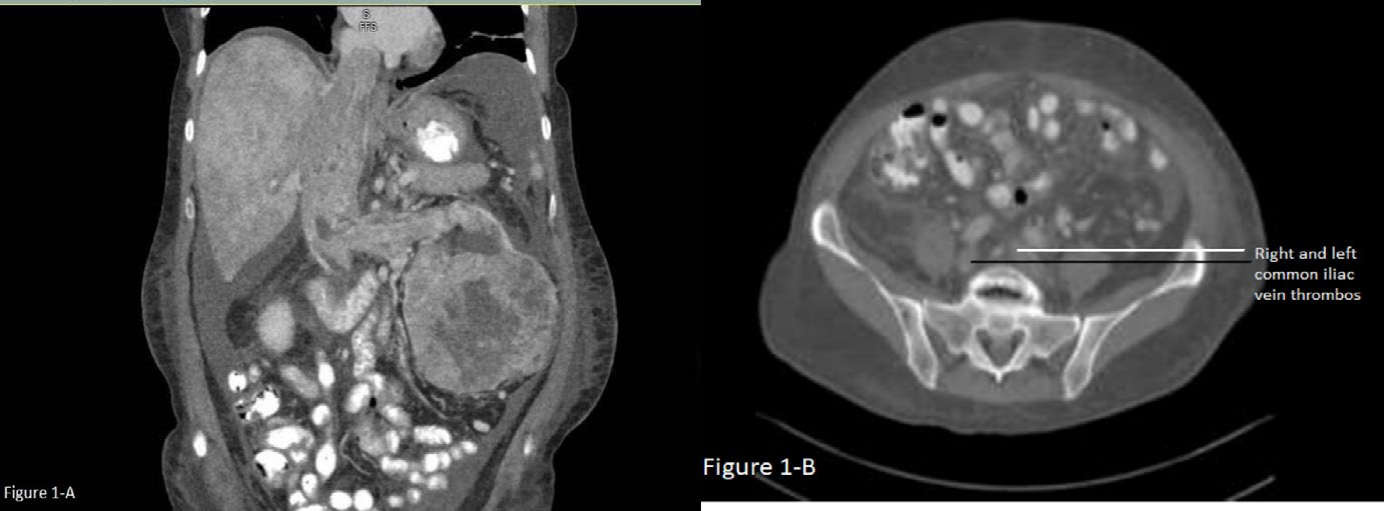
Computed tomography results of abdomen and pelvis showing left renal mass. 1A: Left kidney mass measuring up to 11.6 cm into 10.7 cm in trans‐axial dimension and up to 10.2 cm craniocaudal, with tumor thrombus involving left renal vein and extending cranially via inferior vena cava (Coronal View) 1B: Caudal extension of tumor thrombus with bilateral common iliac veins involvement (Cross‐sectional view)
